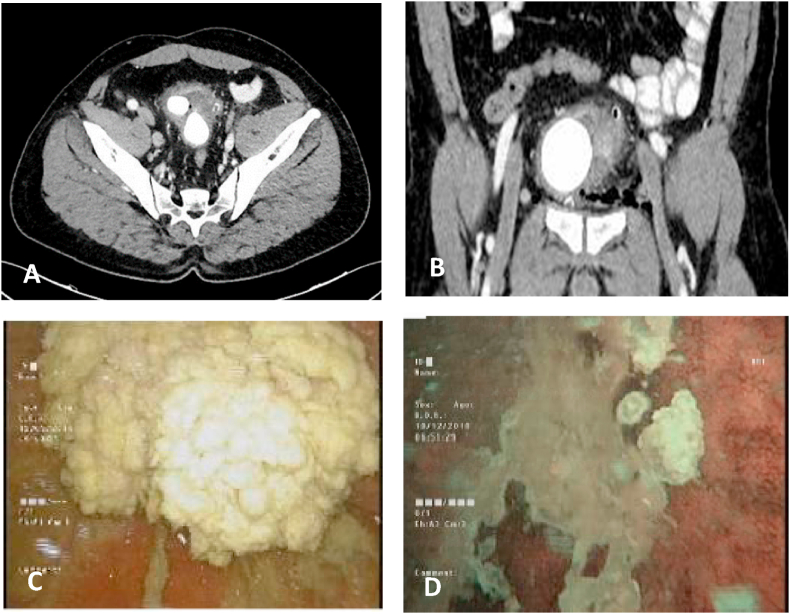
peri-operative CT scan and cystoscopy, A and B Ct scan of urinary bladder stone with a mass arising from anterior urinary bladder wall (axial and coronal views), C: first diagnostic cystoscopy showing extensive whitish exophytic cauliflower lesion, D:follow up cystoscopy showing whitish exophytic cauliflower lesion at the side of the primary tumor.
